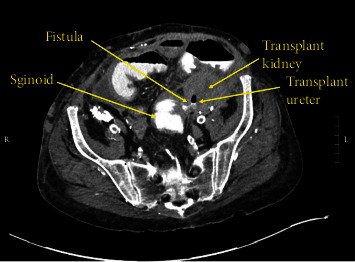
Axial CT of the abdomen and pelvis with rectal contrast demonstrating fistulous connection between the sigmoid colon and left renal transplant ureter.
